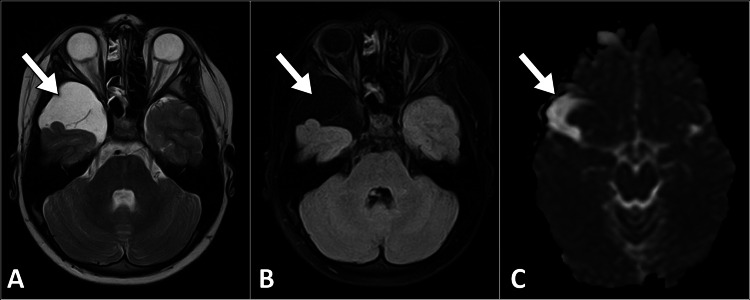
Axial brain MRI images show a lesion in the right middle cranial fossa (arrow) that demonstrates high signal intensity on the T2-weighted image (A) with complete suppression on the FLAIR image (B) and facilitated diffusion on the ADC map (C) These findings are consistent with an arachnoid cyst. MRI: magnetic resonance imaging; FLAIR: fluid-attenuation inversion recovery; ADC: apparent diffusion coefficient
